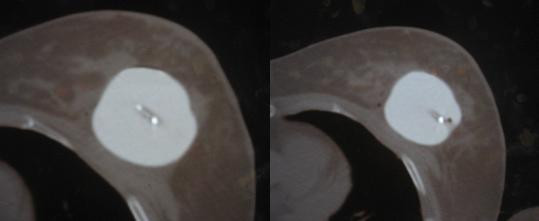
CT scan slices from case 3 showing the asymmetry of the balloon.
